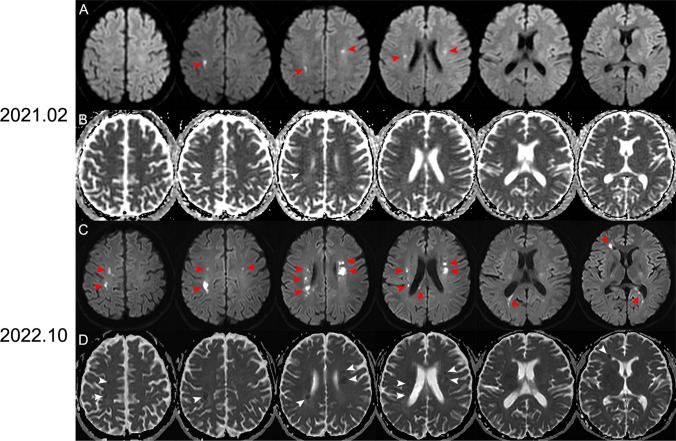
Persistent and deteriorated diffusion restriction dots in deep frontoparietal and periventricular white matter. DWI images (A, C red arrows) showed persistent and deteriorated deep frontoparietal and periventricular white matter diffusion restriction dots, with corresponding reduced ADC (B, D white arrowhead)
